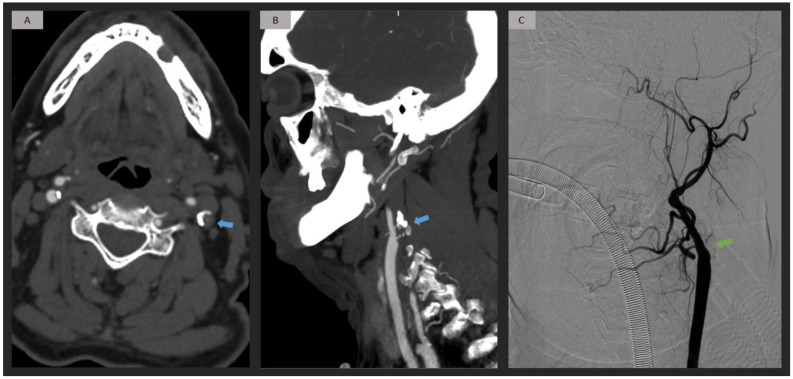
Patient with left origin ICA occlusion attributed to an atherosclerotic plaque. Axial and sagittal CTA images (A,B) exhibit a well-delimited occlusion at the origin of the ICA, resulting from an atherosclerotic plaque with calcifications (blue arrow). DSA confirms the occlusion of the ICA from its origin (green arrow). (C). ICA = internal carotid artery; CTA = computed tomography angiography; DSA = digital subtraction angiography.
